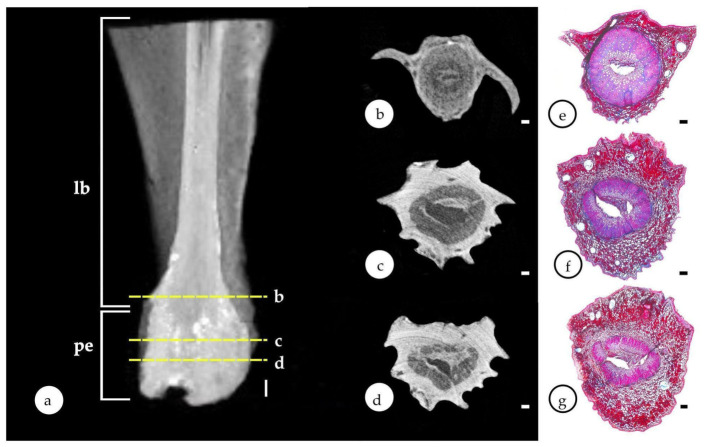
Micro–computed tomography images and anatomical characterization of petiole in *Hortia oreadica*. (a) General view of the petiole. (b–d) Showing the vascular cylinder closed along the petiole. (e–g) Sections transversal showing the vascular cylinder closed along the petiole. lb = leaf blade; pe = petiole. Scale bars: (a) = 100 μm; (b–g) = 200 μm.
